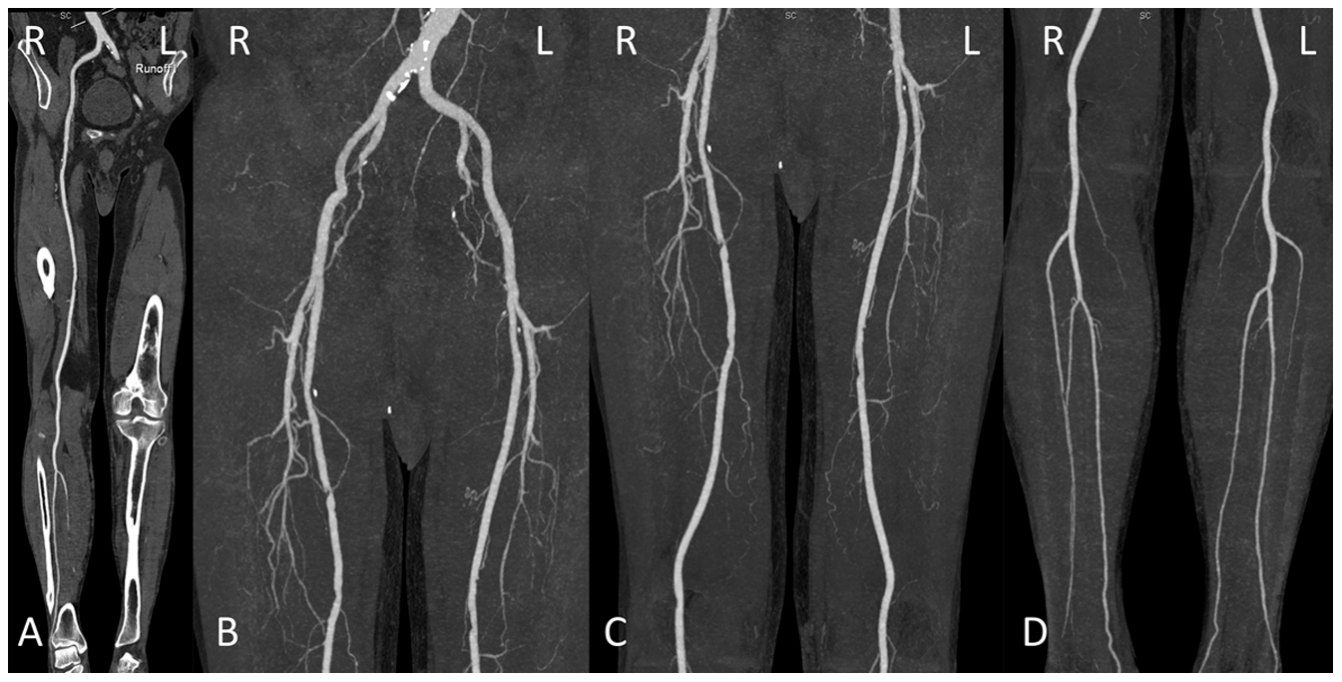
Lower extremity CTA using 70-year-old man with PAD. A, curved planar reformatted image and B–D, maximum intensity projection images. The image quality was visually classified as score 4 (excellent) in the aortoiliac (B), femoropopliteal (C) and lower leg segments (D).
